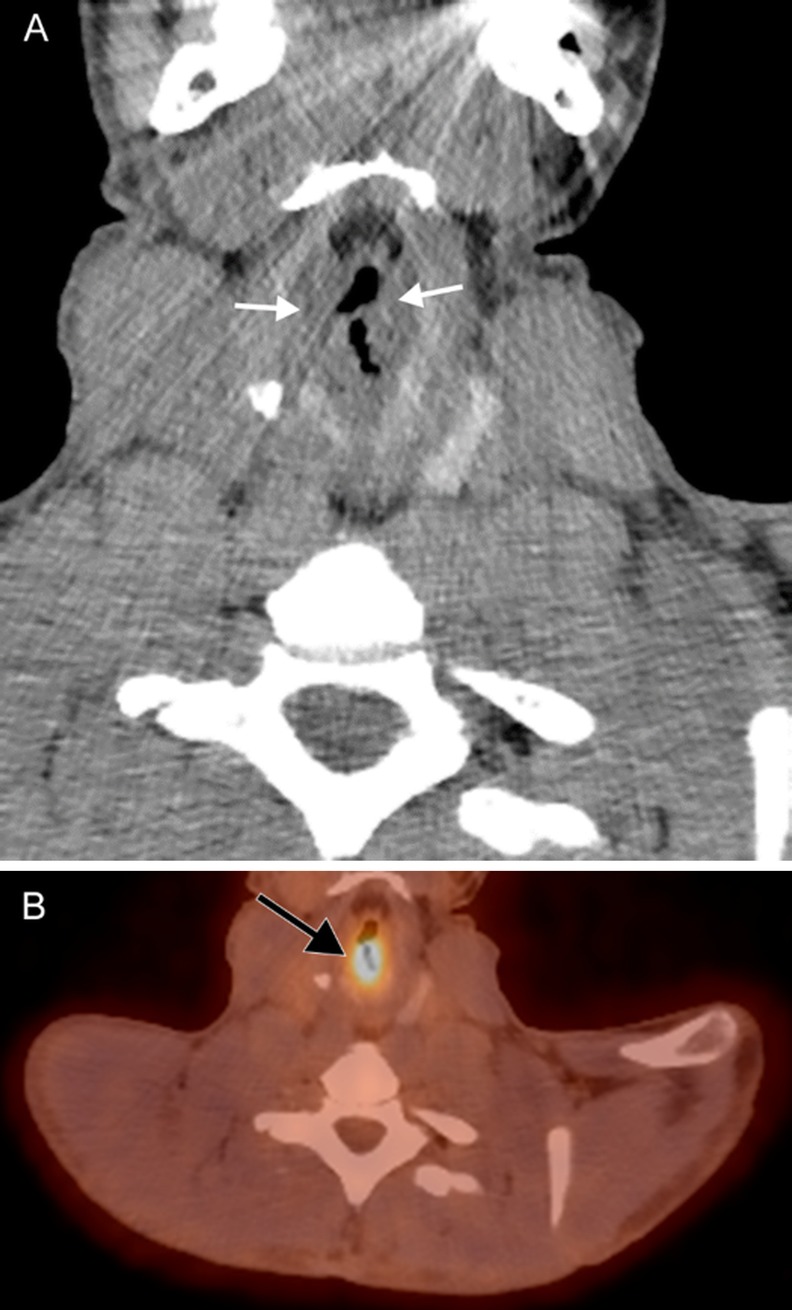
Laryngeal papillomatosis. A 26-year-old man with recurrent tracheal/laryngeal papillomatosis status post tracheal diversion and radiation therapy. A coronal contrast-enhanced CT image (a) reveals nodular soft tissue attenuation (white arrows) replacing the laryngeal structures. Subsequent axial attenuation-corrected PET image (b) reveals marked FDG-avidity (black arrow)
